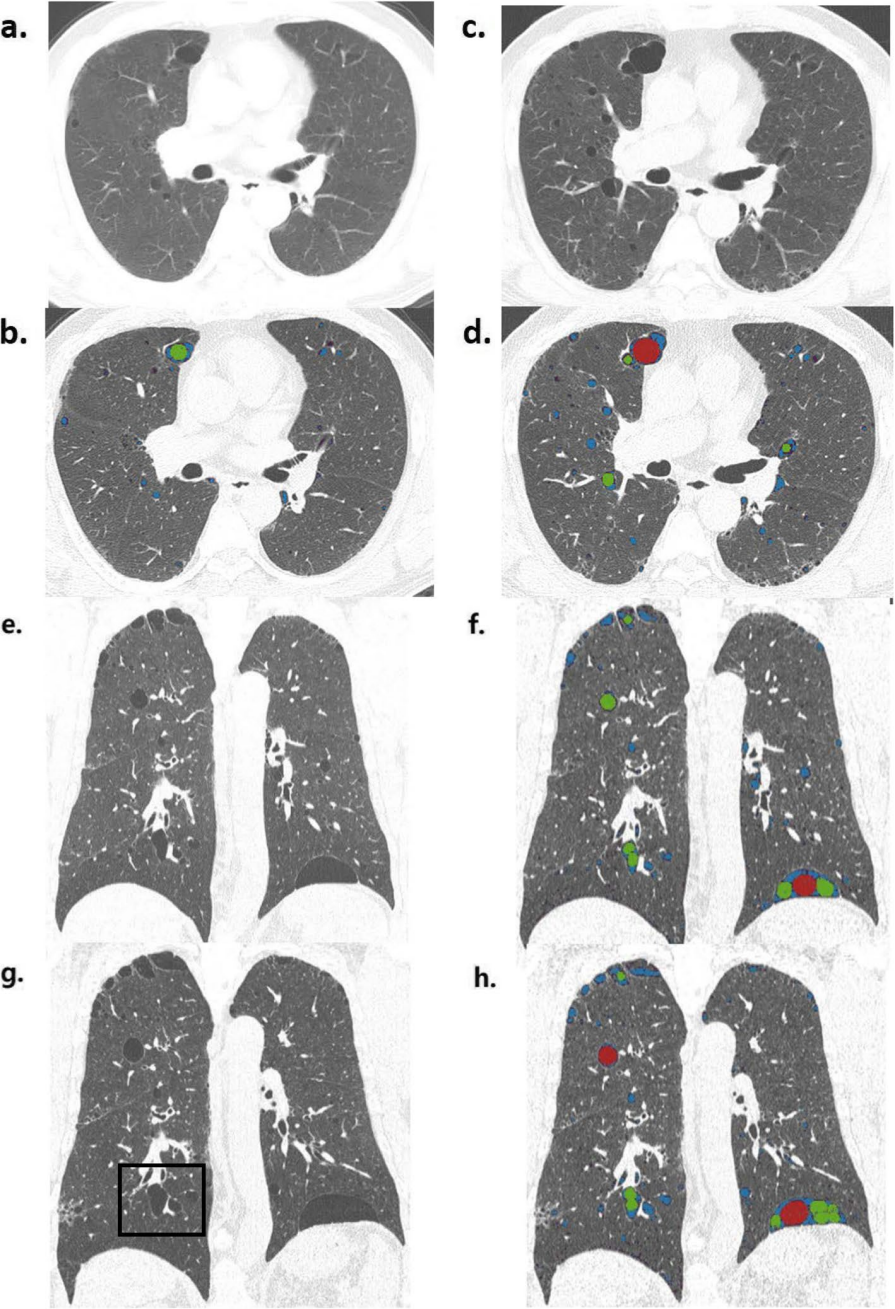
A representative case showing cyst progression in a 54-year-old male with BHD disease. a, b, e, f Baseline axial and coronal CT images and figures. c, d, g, h CT images obtained 6 years later. b, d, f, h Low attenuation area as a color map according to cyst size analyzed by in-house software. Black box in g indicates a cyst with “air-cuff signs”
